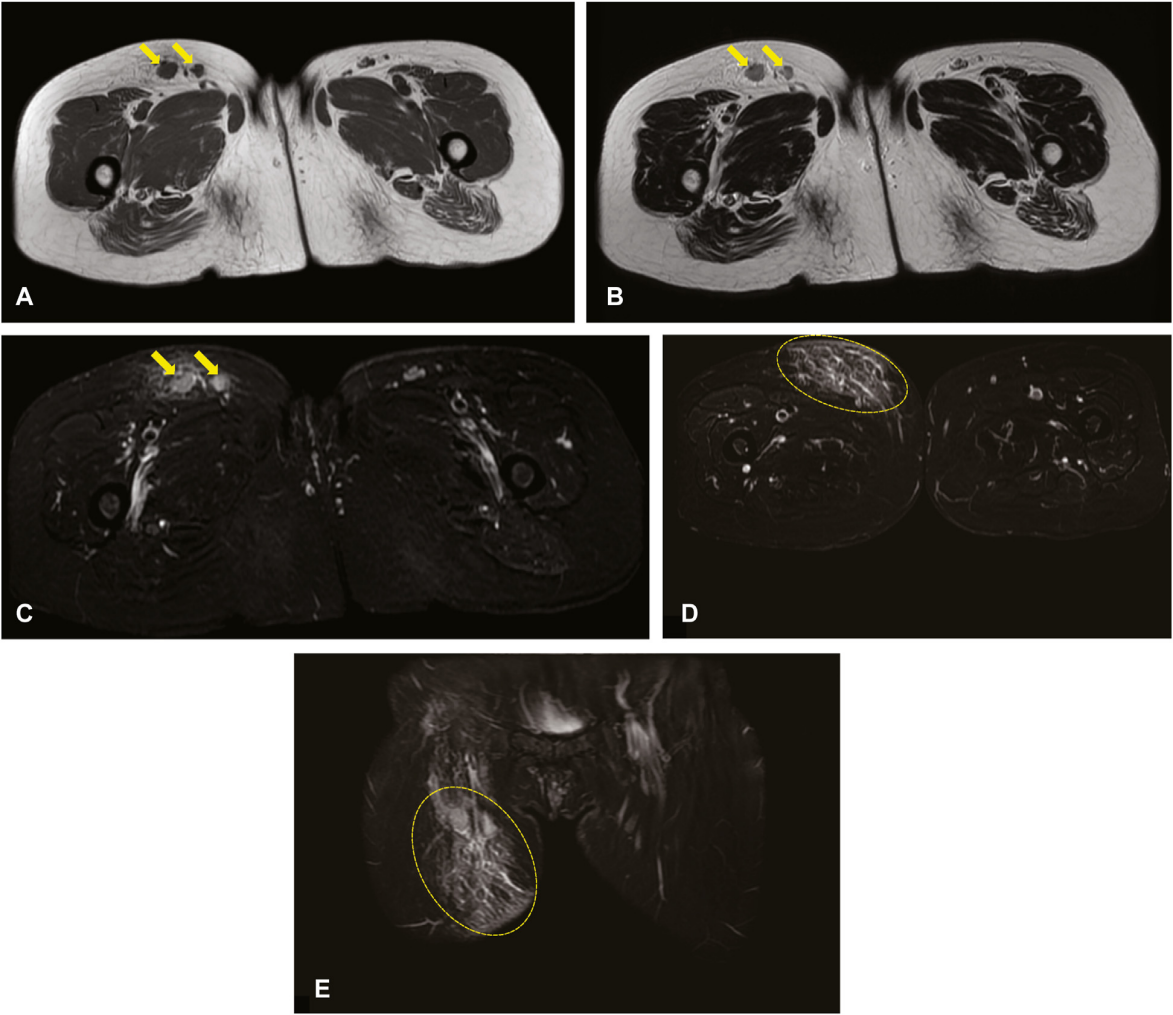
Imaging findings of the patient. Magnetic resonance imaging (MRI) reveals several lymph nodes in the right inguinal region. A, Transverse T1-weighted magnetic resonance imaging shows a homogeneous tumor with high signal intensity *(yellow arrows*). B, Transverse T2-weighted magnetic resonance imaging shows a heterogeneous tumor with high signal intensity (*yellow arrows*). C, The tumor region of interest is delineated on a diffusion-weighted image (*yellow arrows*) and (D) mirrored to an apparent diffusion coefficient map (*yellow oval*), which is derived using b-values of 0 and 1000 s/mm^2^. E, The corresponding apparent diffusion coefficient histogram of the whole tumor volume demonstrates a rightward shift (*yellow oval*) (apparent diffusion coefficient values [31,023 mm^2^/s]: mean, 1.76; 10th percentile, 1.33; 25th percentile, 1.51; 50th percentile, 1.75; 75th percentile, 2.02; 90th percentile, 2.20, Coefficient of Variation; 0.19; skewness; −0.05).
